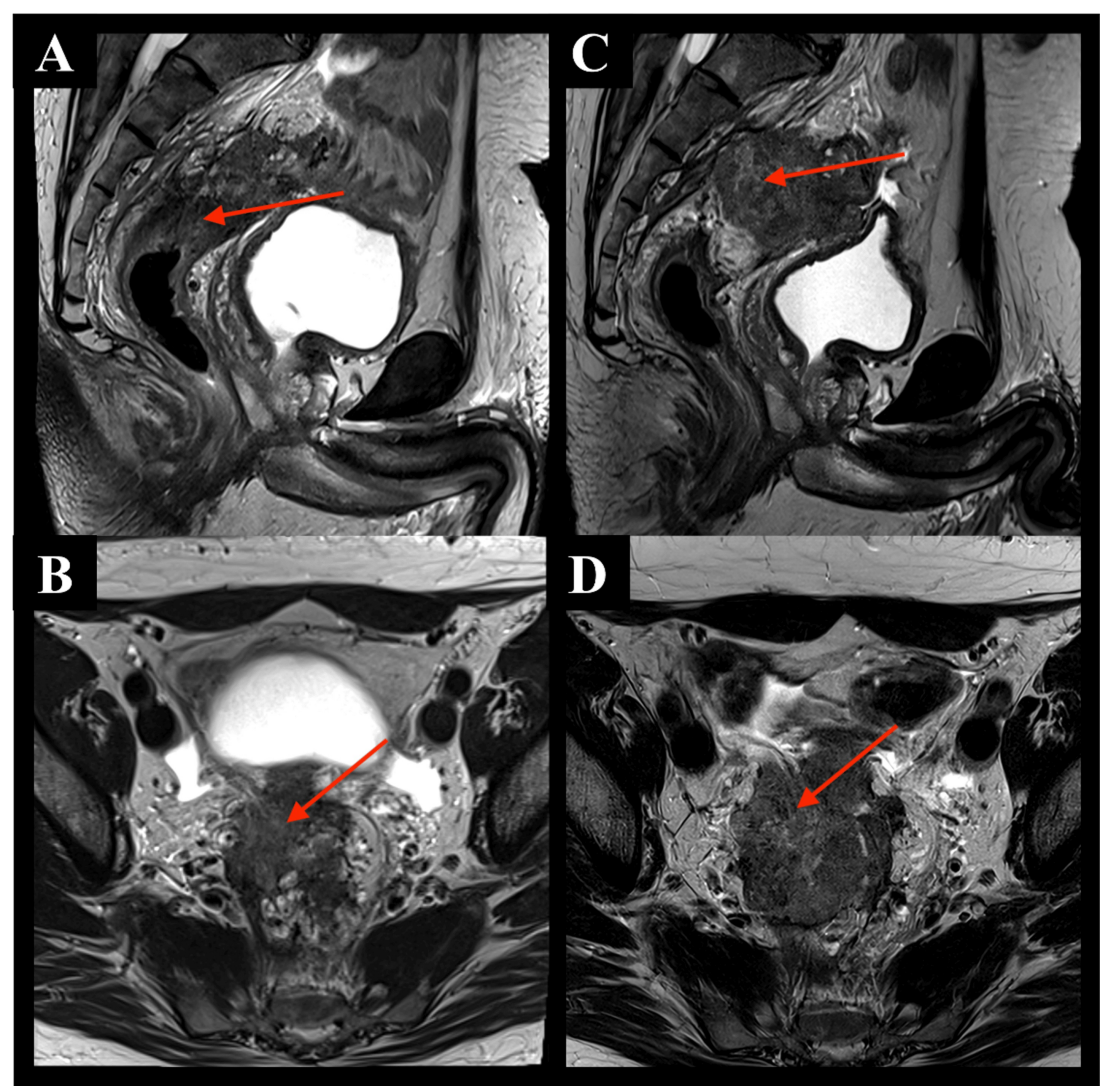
Restaging MRI after chemotherapy showing the rectal tumor 8.5 cm from the anal verge and 6.4 cm in craniocaudal length and pelvic MRI for radiation treatment planning demonstrating tumor progression, 6.8 cm in craniocaudal length. A: Restaging MRI sagittal view; B: Restaging MRI axial view; C: Radiation treatment planning MRI sagittal view; D: Radiation treatment planning MRI axial view. Red arrows indicate the primary tumor.
